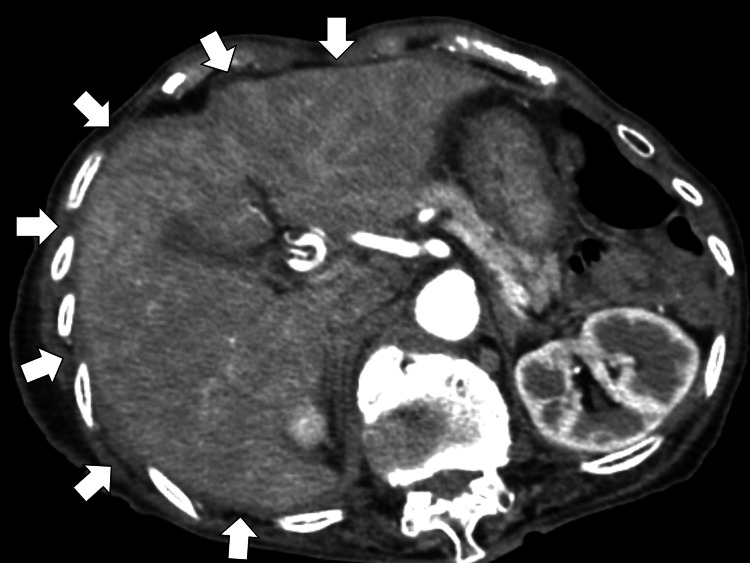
Abdominal contrast-enhanced computed tomography showing the mottled contrast effect of the liver without dilatation of the cholangial duct (white arrows)
